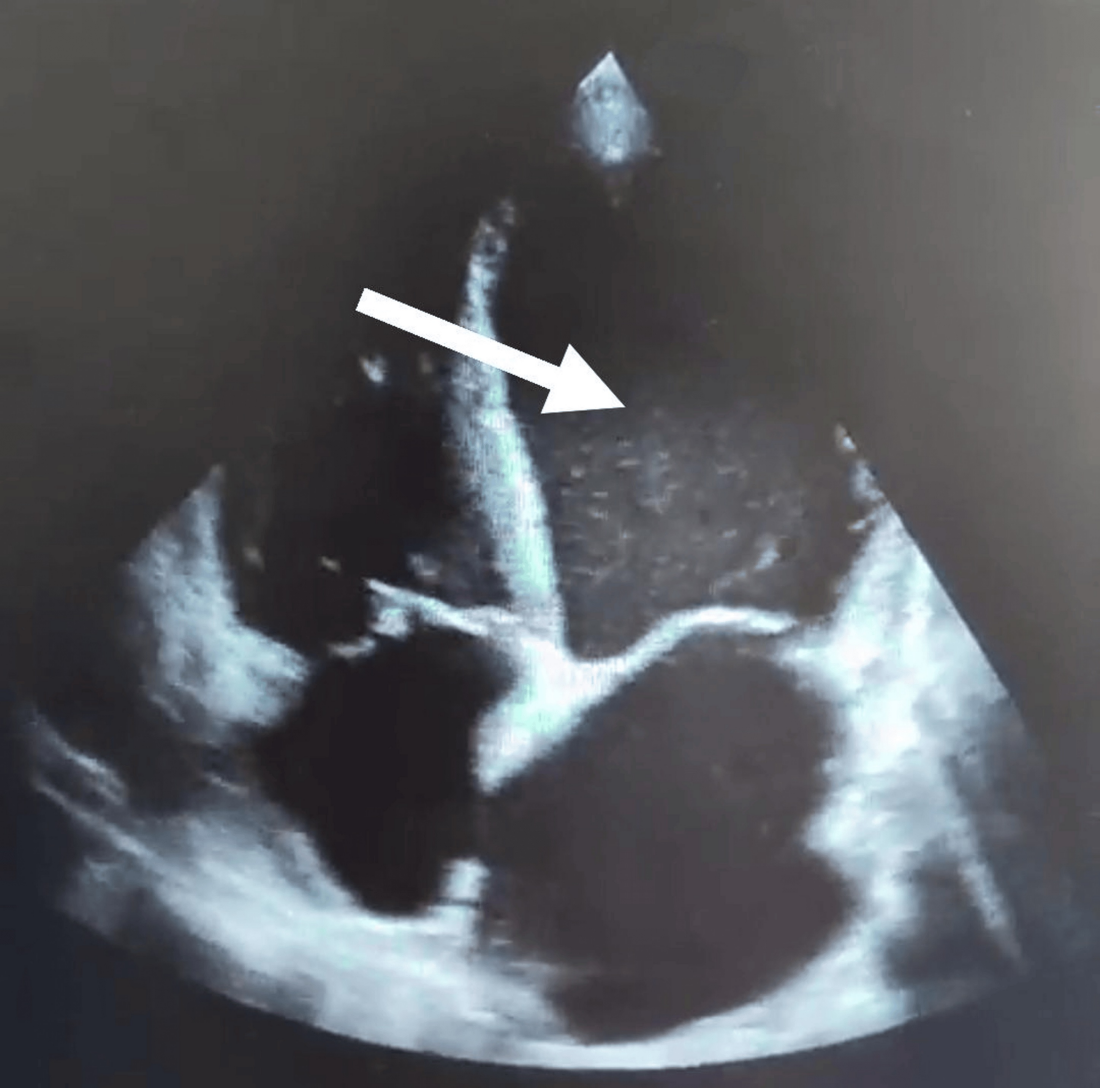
Initial transthoracic echocardiography revealing dilated heart chambers with reduced ejection fraction (30%).
